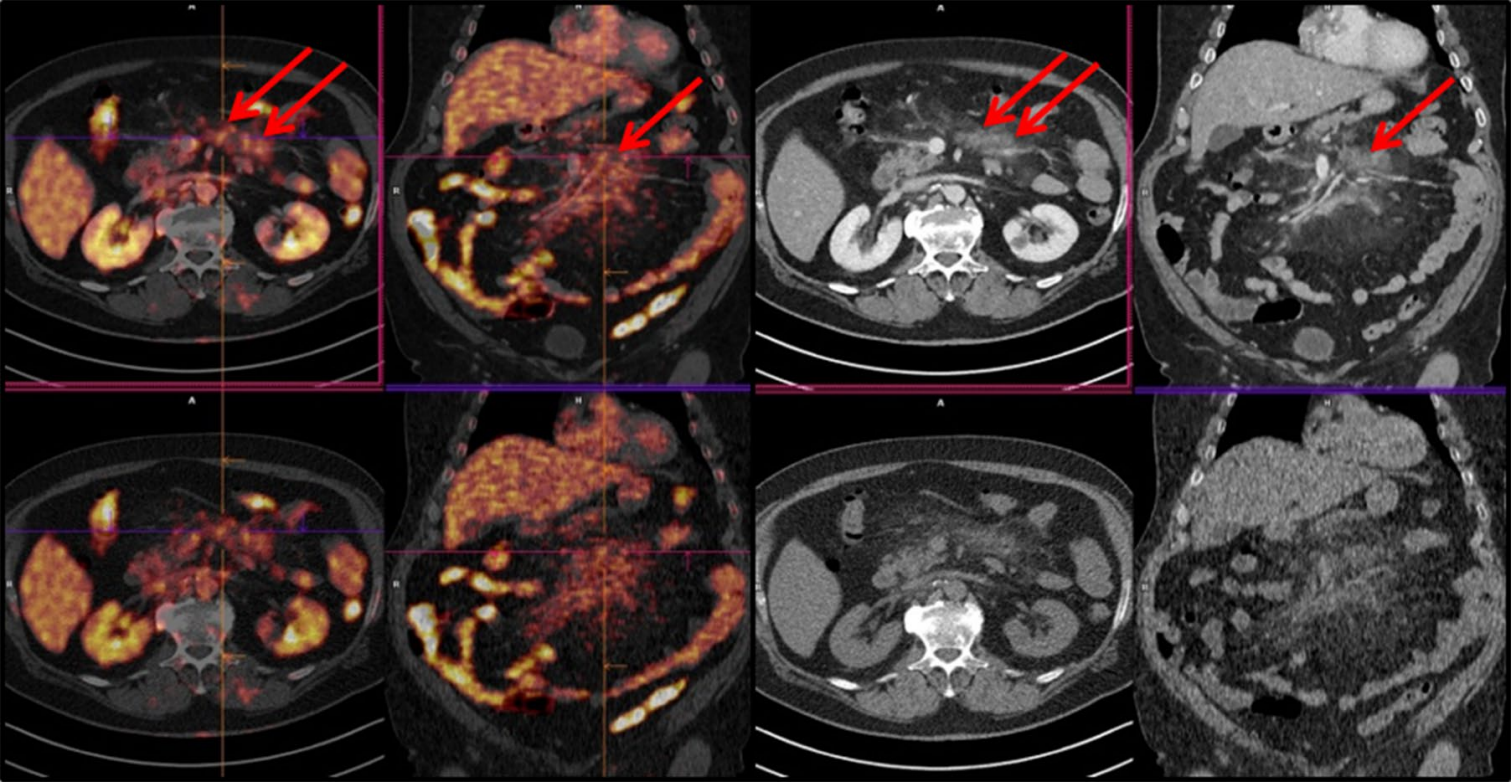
PET/ceCT highlighted additional lesions in mesenteric area [DS 4; SUVmax 4.1].
